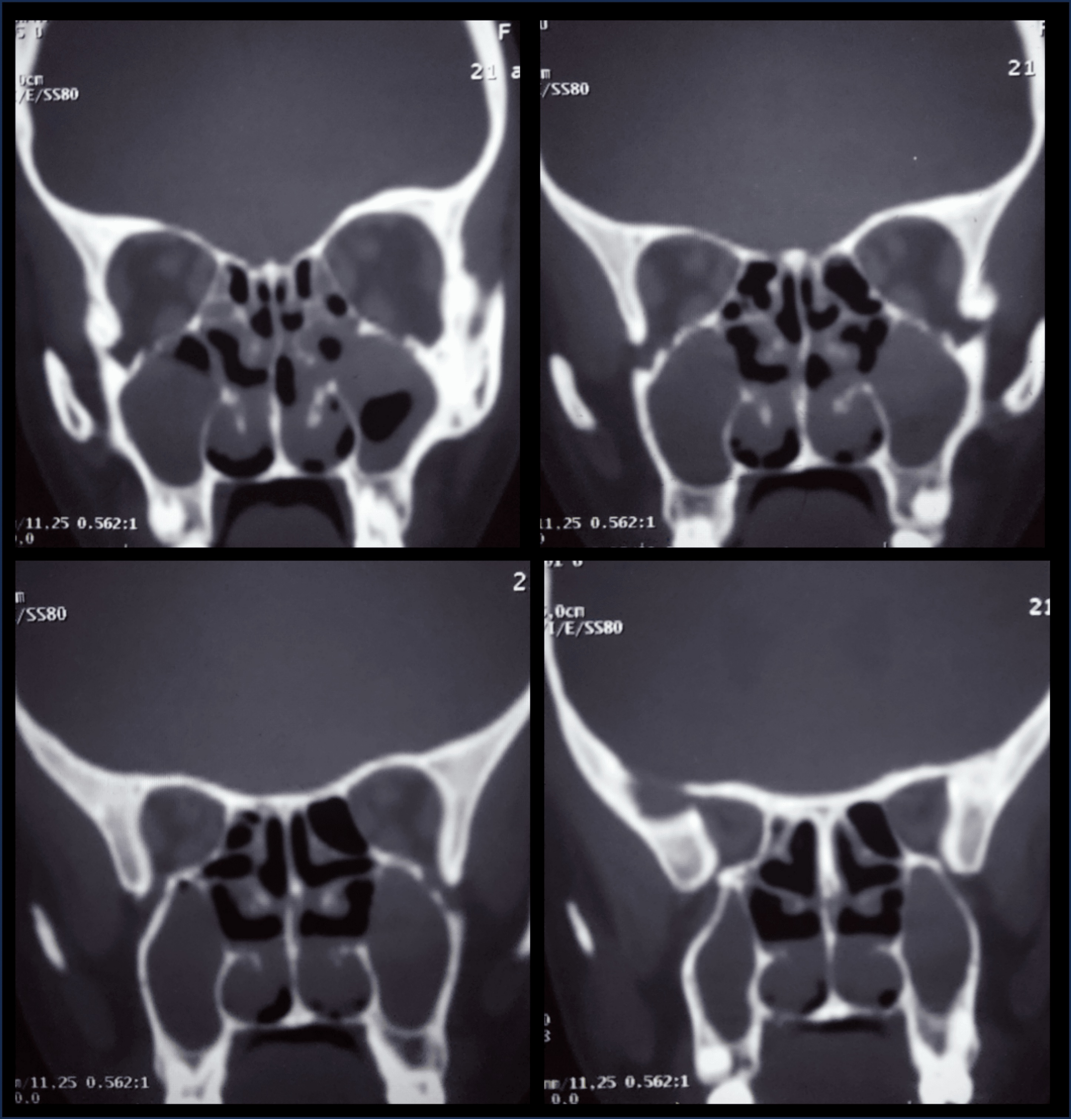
Sinus CT scan showed acute pansinusitis on a background of chronic sinus disease.
